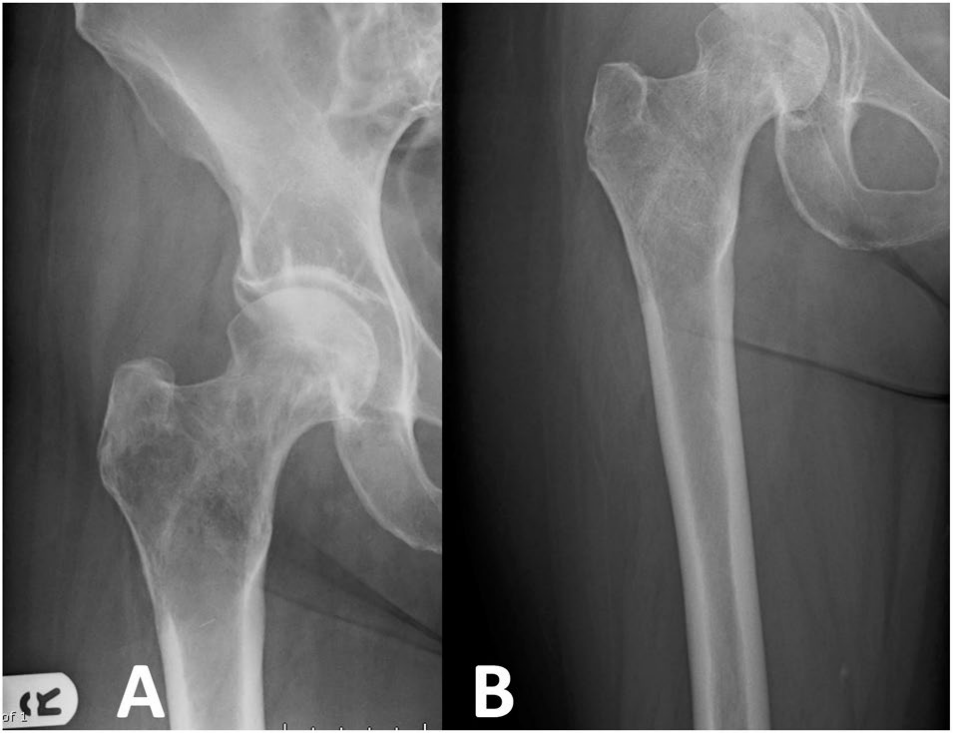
A patient at first presentation with metastatic breast cancer and a large lucent lesion in the peritrochanteric region and a Mirels score of ten (A). She was treated with denosumab, tamoxifen and radiotherapy. Follow up radiographs (B) showed the lesion had ossified and symptoms improved with medical management alone and surgical treatment was not required.
